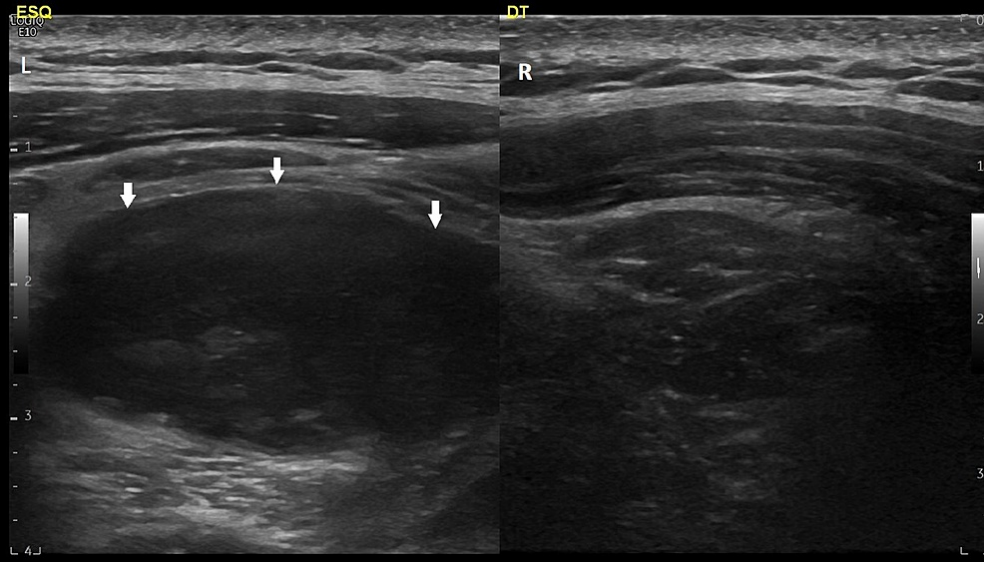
Heterogeneous collection (arrows) seen in ultrasound From: Ana Sofia Costa, Department of Radiology, Hospital Prof. Doutor Fernando Fonseca
